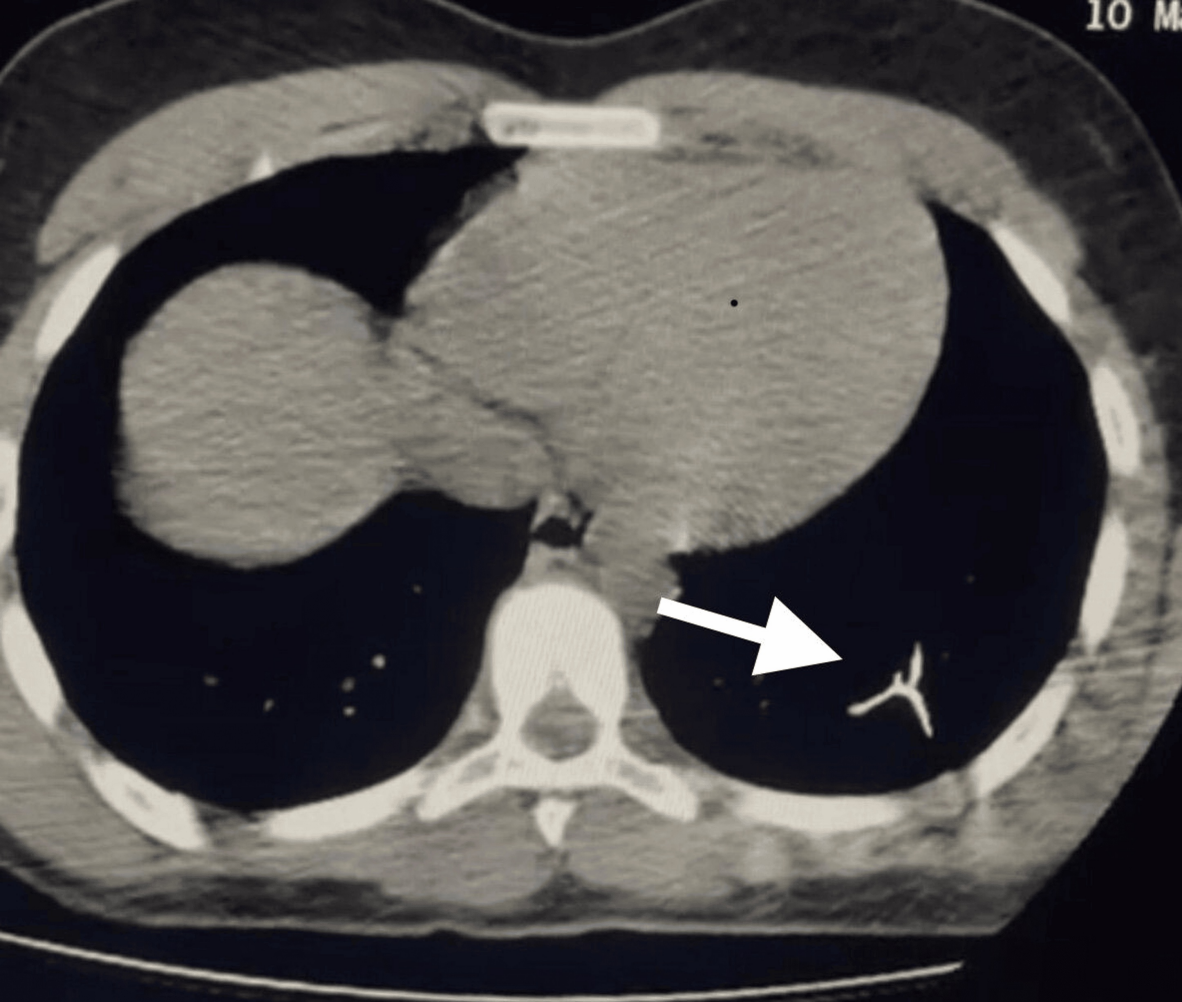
Chest CT scan showing a FB in the left basal lung (10/05/2024) FB, foreign body
